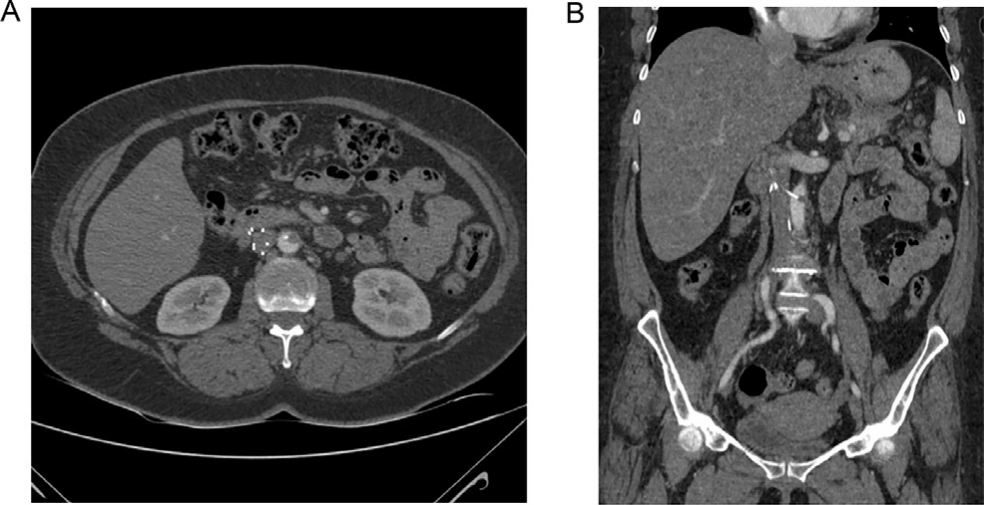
(A) Axial contrast-enhanced CT scan of the abdominal and pelvic cavities, which reported that at least three struts of the IVC filter were out of the IVC (blue arrow) and one strut was penetrated into the abdominal Aorta (red arrow). A small crescent-shaped hyperdense area is visible at the point of contact, consistent with partial aortic thrombosis. (B) Coronal contrast-enhanced CT scan demonstrating the position of the IVC filter (red arrow) and the proximity of one strut to the aortic wall. A focal crescentic hyper density at the tip of the strut (orange arrow) is consistent with partial aortic thrombosis. (yellow arrow).
